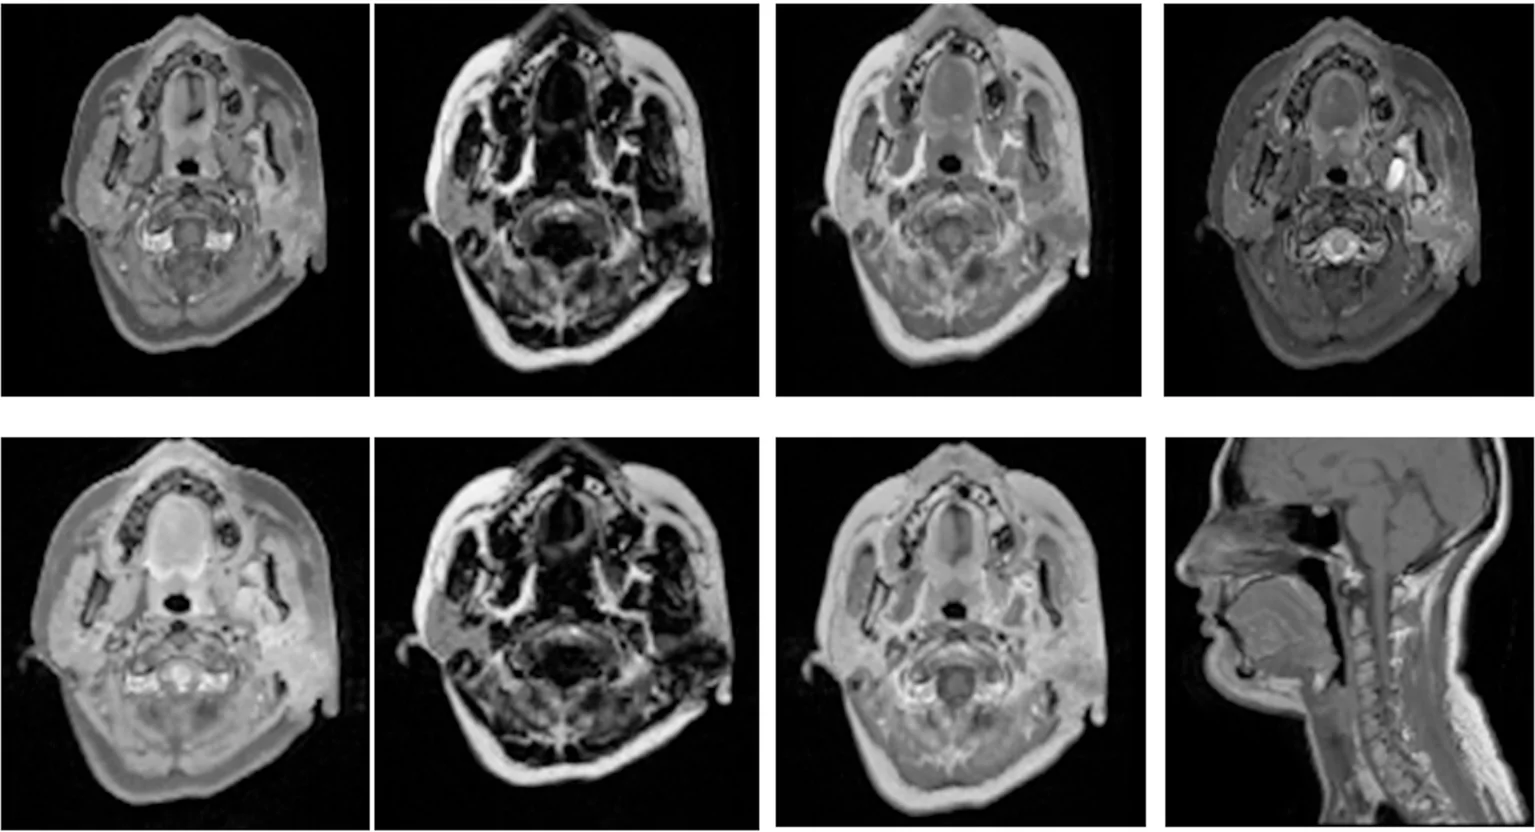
MRI demonstrating a left-sided infiltrative facial neurofibroma. Axial and sagittal MRI sequences show a heterogeneous soft-tissue mass involving the left parotid and adjacent facial spaces, with features consistent with neurofibroma.
